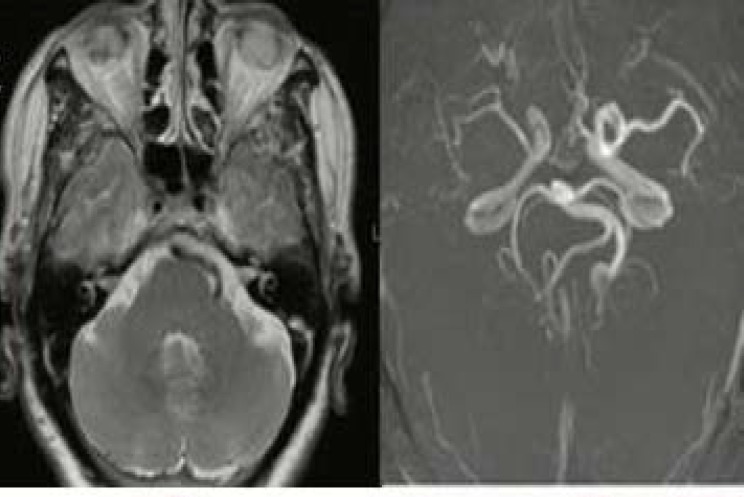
Magnetic resonance imaging (MRI) and angiography revealed dolichoectasia of distal left internal carotid artery and left vertebral and basilar arteries, but no apparent aneurysm
